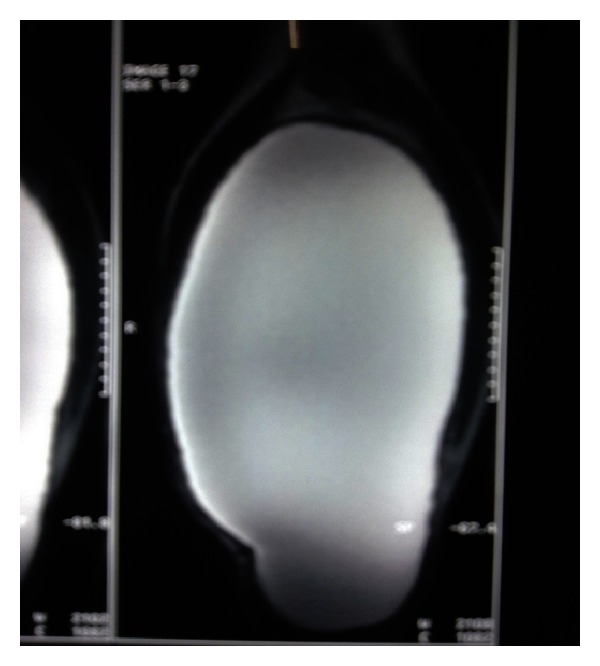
MRI right lower limb showing large cystic mass.
